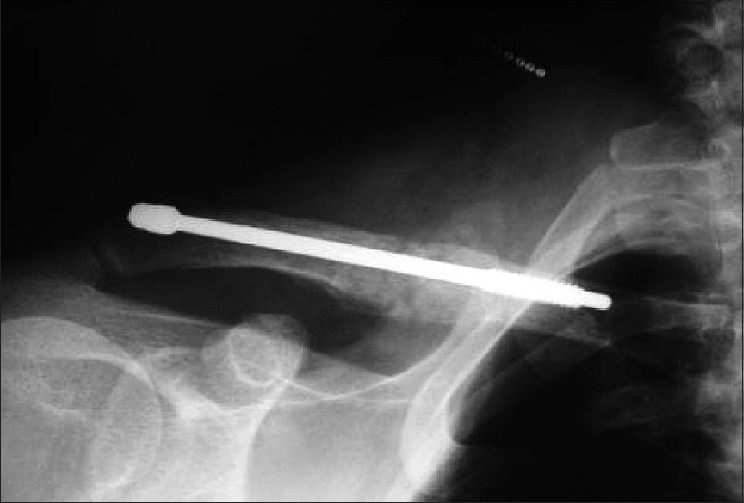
Radiograph showing intramedullary stabilization using rockwood clavicle pins
